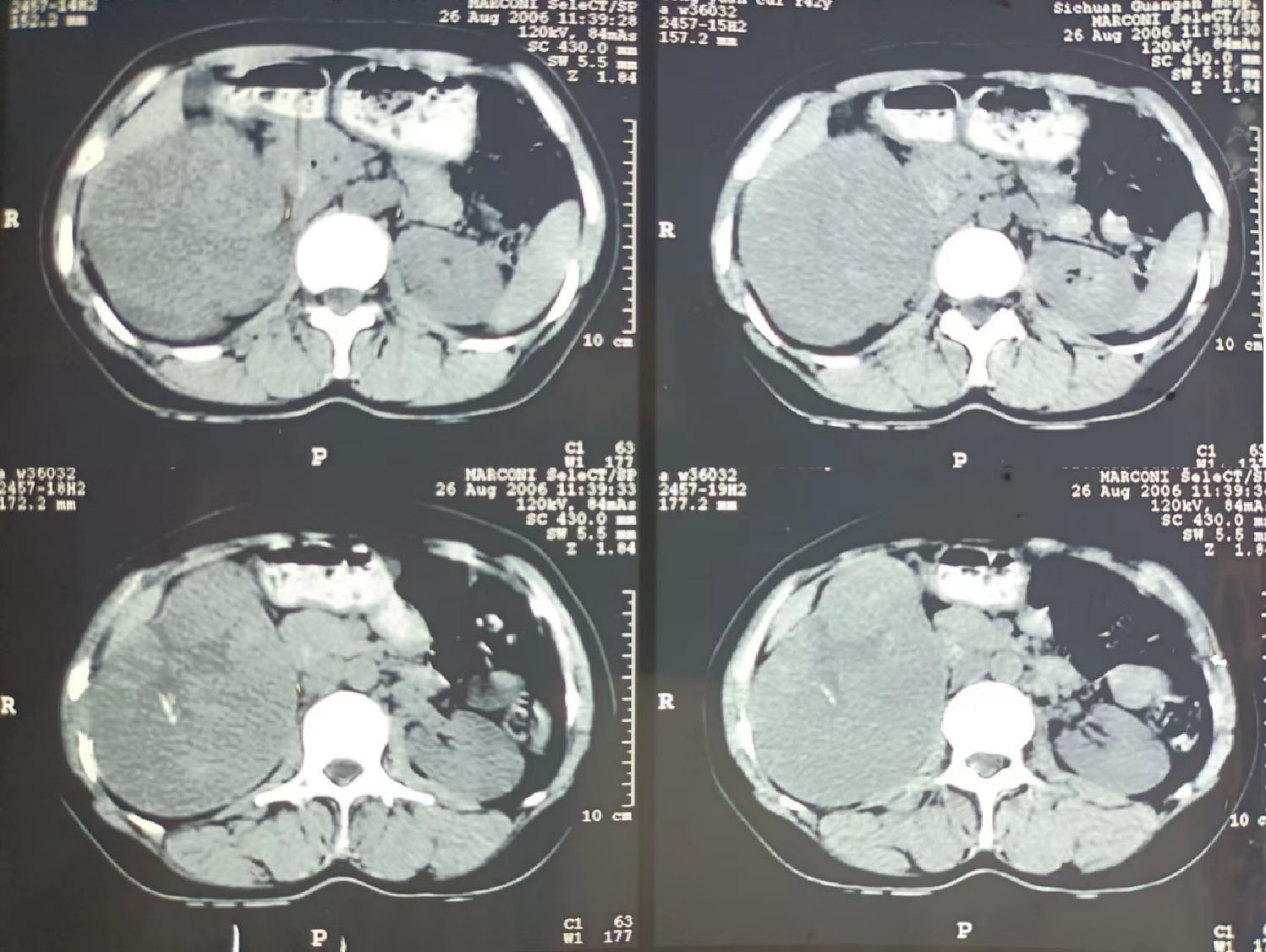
A computed tomography (CT) scan conducted 17 years ago revealing a mass with a maximum cross-sectional diameter of 13 × 8 cm in the right kidney is shown. Several calcifications within the tumor are observable.
